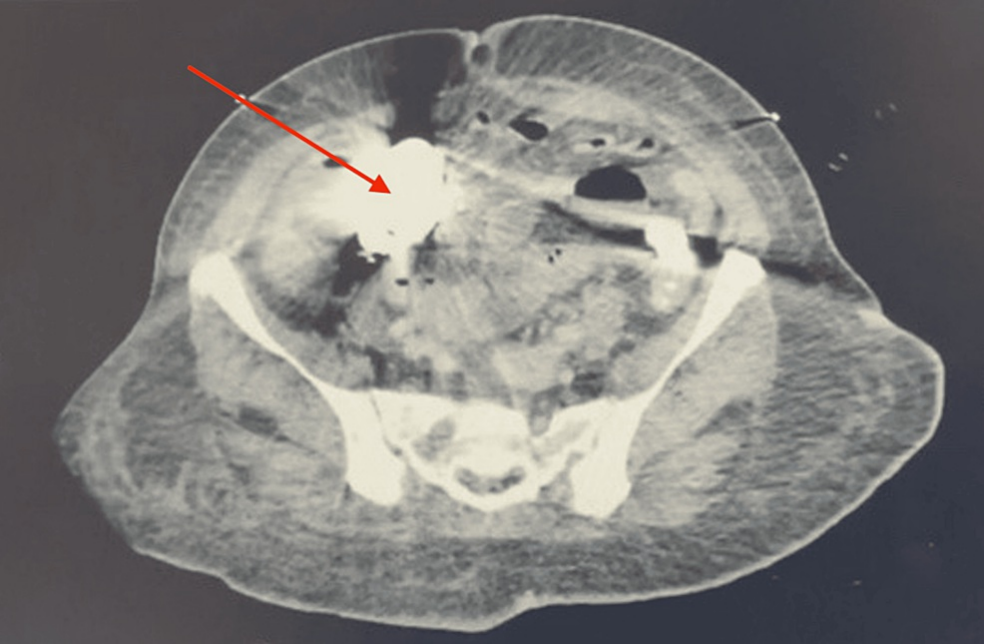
Axial CT of abdomen and pelvis with/without contrast showing barium impaction (red arrow) in sigmoid colon CT: Computed tomography
